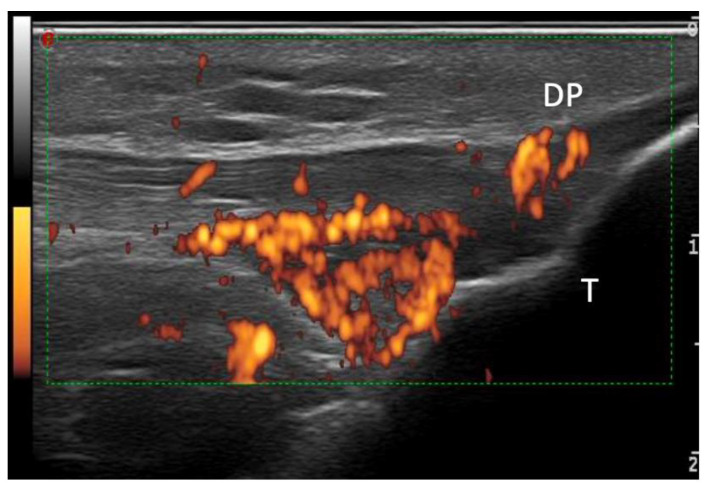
Power Doppler US image of the patellar tendon’s distal enthesis, showing a hypoechoic tendon, with the loss of a homogenous fibrillar pattern primarily near the cortical bone (<2 mm), with an intense power Doppler signal. DP—distal patellar tendon; T—tibial tuberosity.
